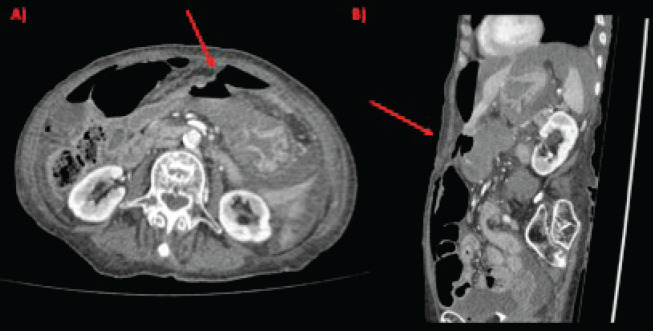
CT scan showing gastric perforation (red arrow) and pneumoperitoneum in (a): axial section and (b): sagittal section.
